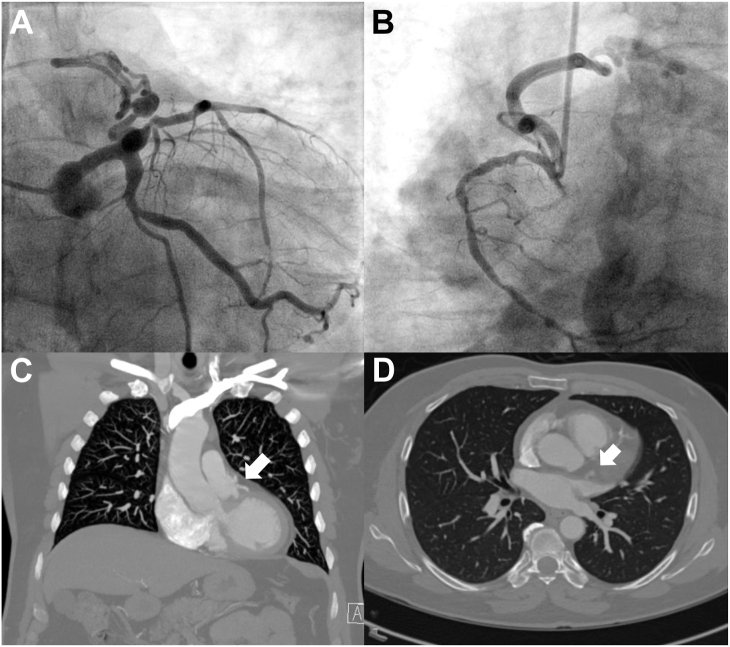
Left coronary angiogram demonstrates fistula formation between the (A) left anterior descending coronary artery and pulmonary artery and (B) fistula formation between the right coronary artery and pulmonary artery. Computed tomography shows fistula feeding into the main pulmonary artery (arrows) with representative (C) coronal and (D) axial images.
